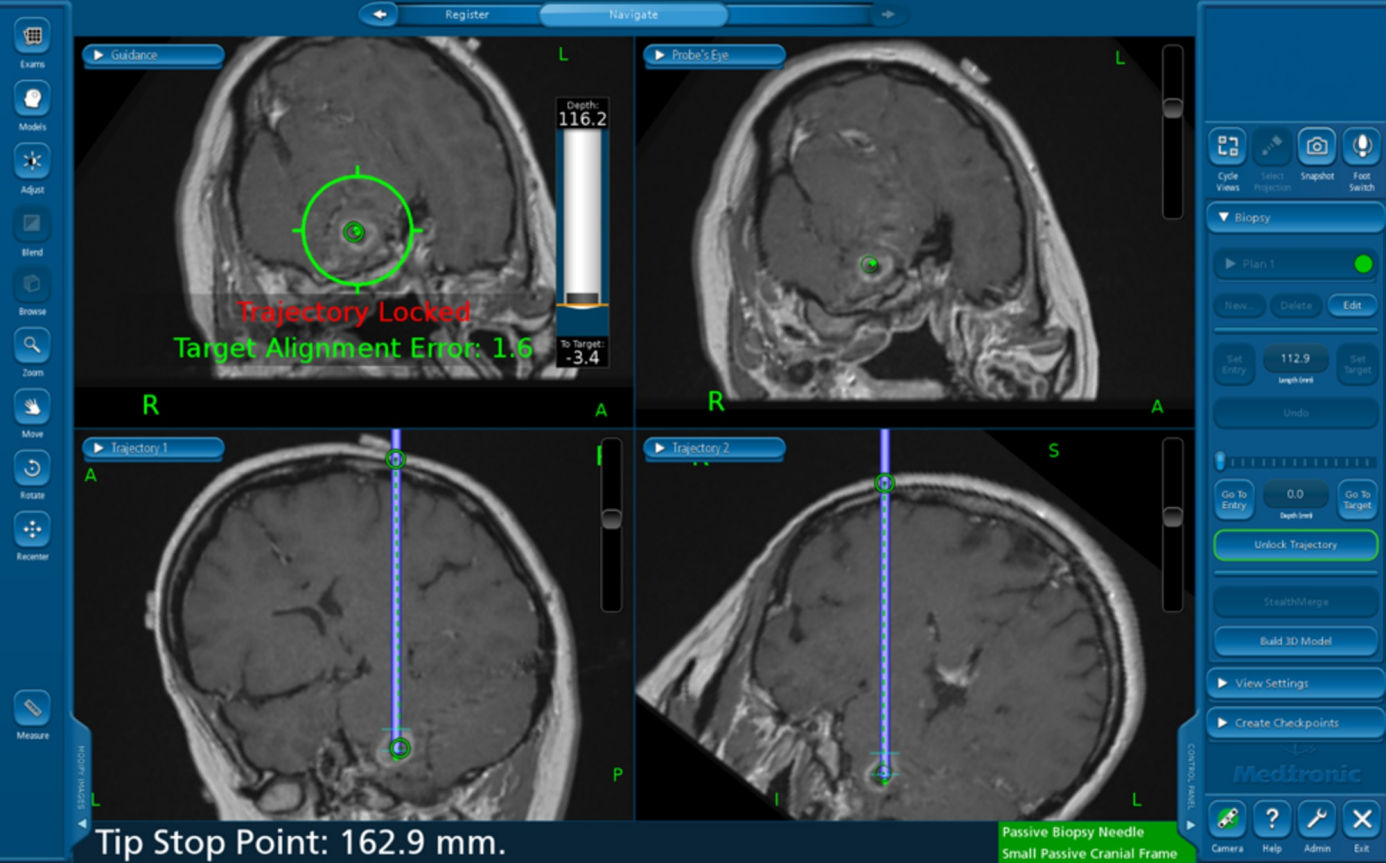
Live image of stealth CT neuronavigation interface during biopsy. Stealth CT neuronavigation allowed surgeons to visualize the anatomy of the patient’s brain during the closed stereotactic needle biopsy. The precise tracking of the location of surgical instruments in relation to the patient’s anatomy and accommodation for brain shift allowed for more precise navigation during surgery. CT - computed tomography.
